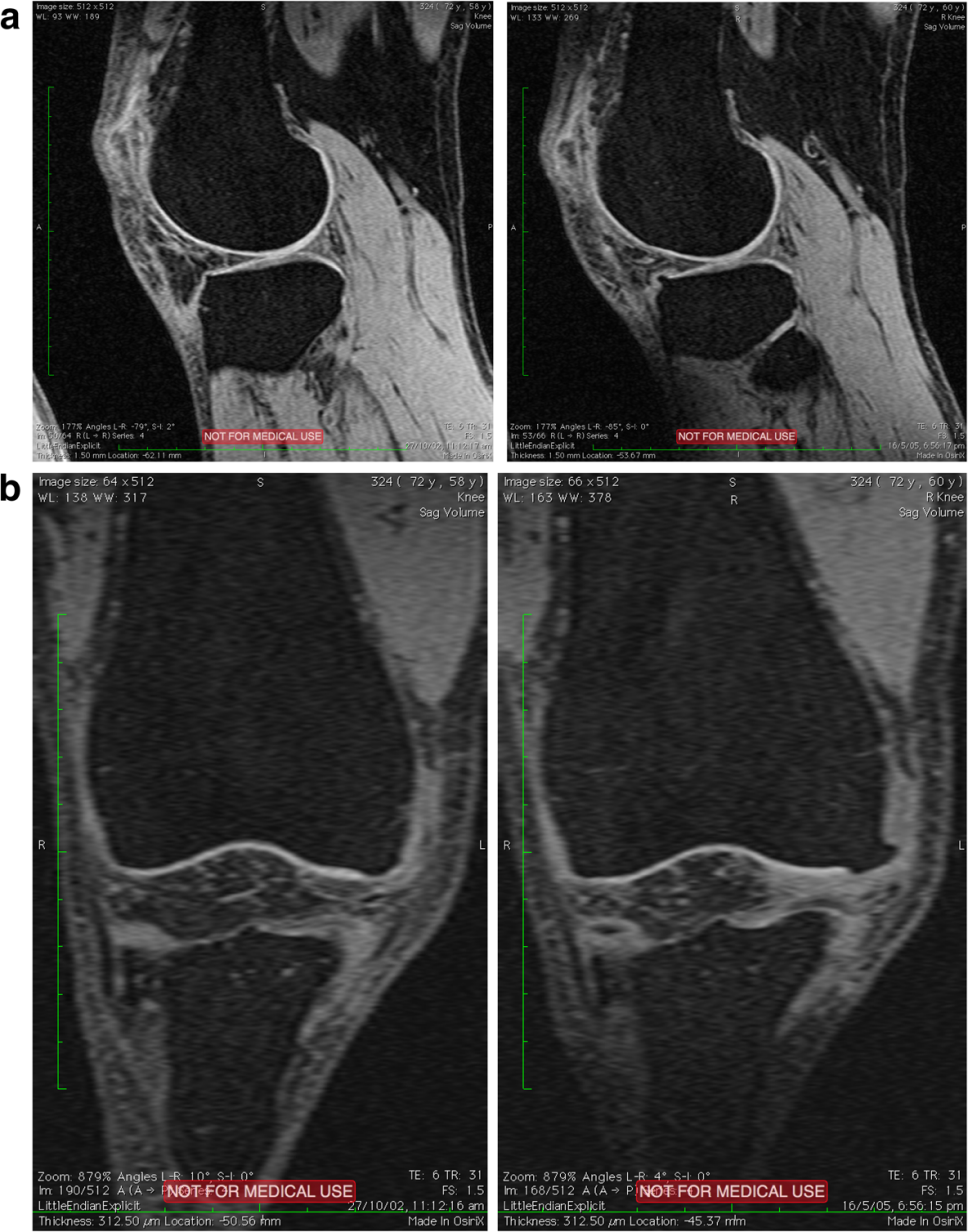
Sample images show magnetic resonance imaging (MRI)-detected osteophyte (OP) progression. a Tibial MRI-detected OP increases from baseline to follow up (wider arrow indicates follow-up MRI-detected OP). b Femoral MRI-detected OP increases from baseline to follow up (wider arrow indicates follow-up MRI-detected OP)
